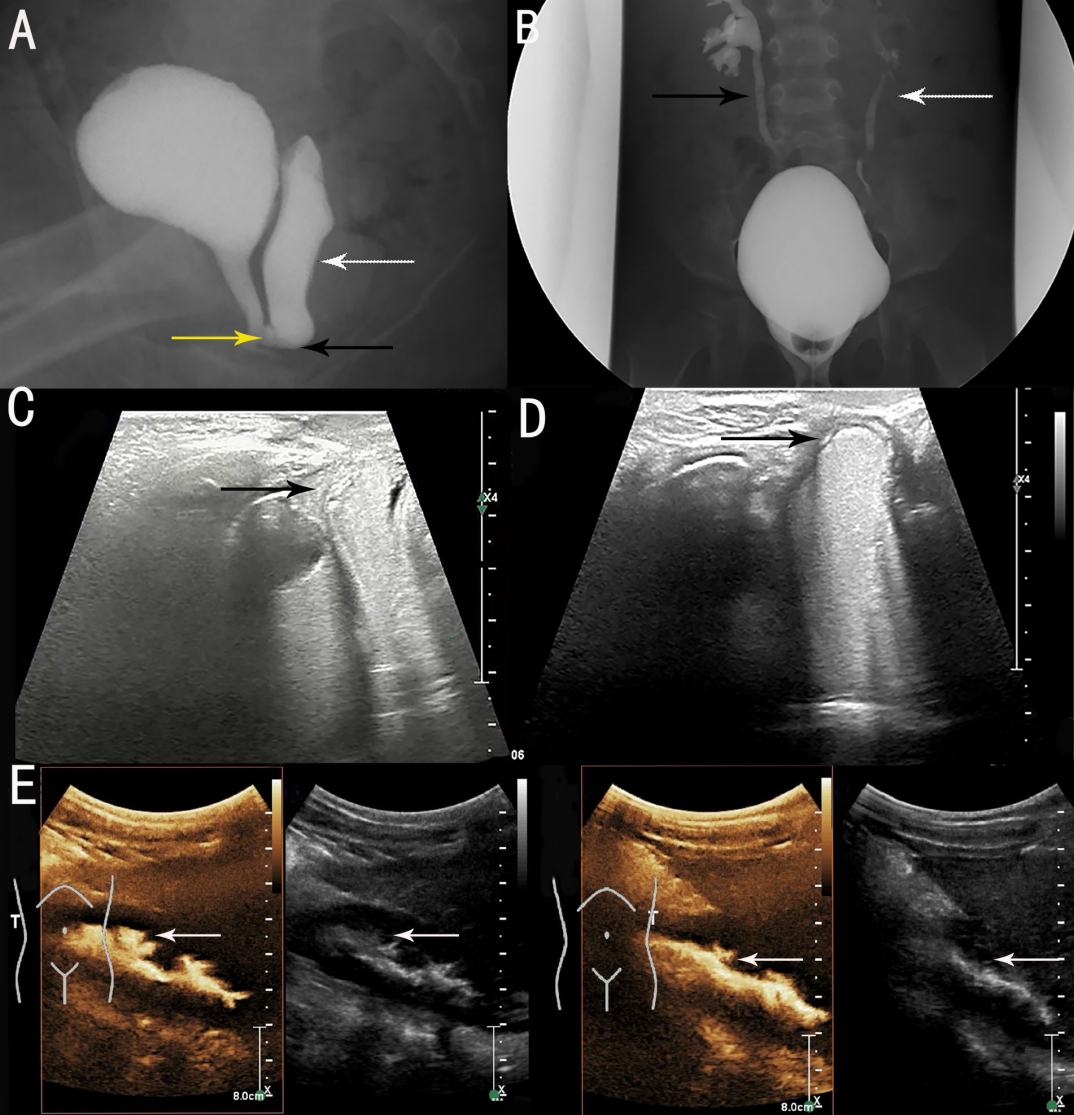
Preoperative imaging examinations. (A) voiding cystourethrography (VCUG) showed vaginal obstruction (black arrow), vaginal effusion (white arrow) and urethra-vaginal fistula (yellow arrow). (B) VCUG showed bilateral VUR (grade III on right side [black arrow] and grade II on left side [white arrow]). (C) contrast-enhanced voiding urosonography (ceVUS) revealed urethra-vaginal fistula (black arrow). (D) ceVUS revealed vaginal obstruction with vaginal effusion (black arrow). (E) ceVUS revealed bilateral VUR (grade III on right side and grade II on left side) (white arrows).
